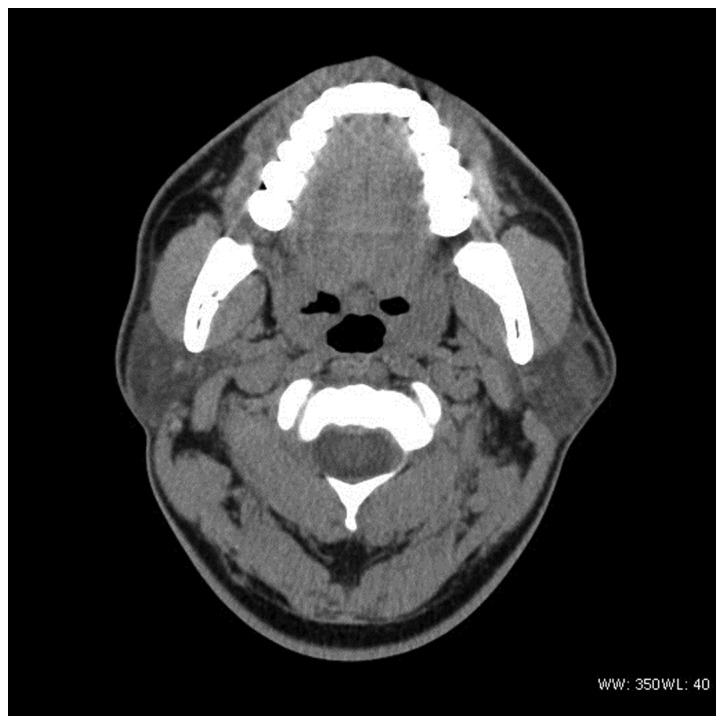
CT plain scan showing an oval, heterogeneous, well-defined mass in the left parotid gland.
